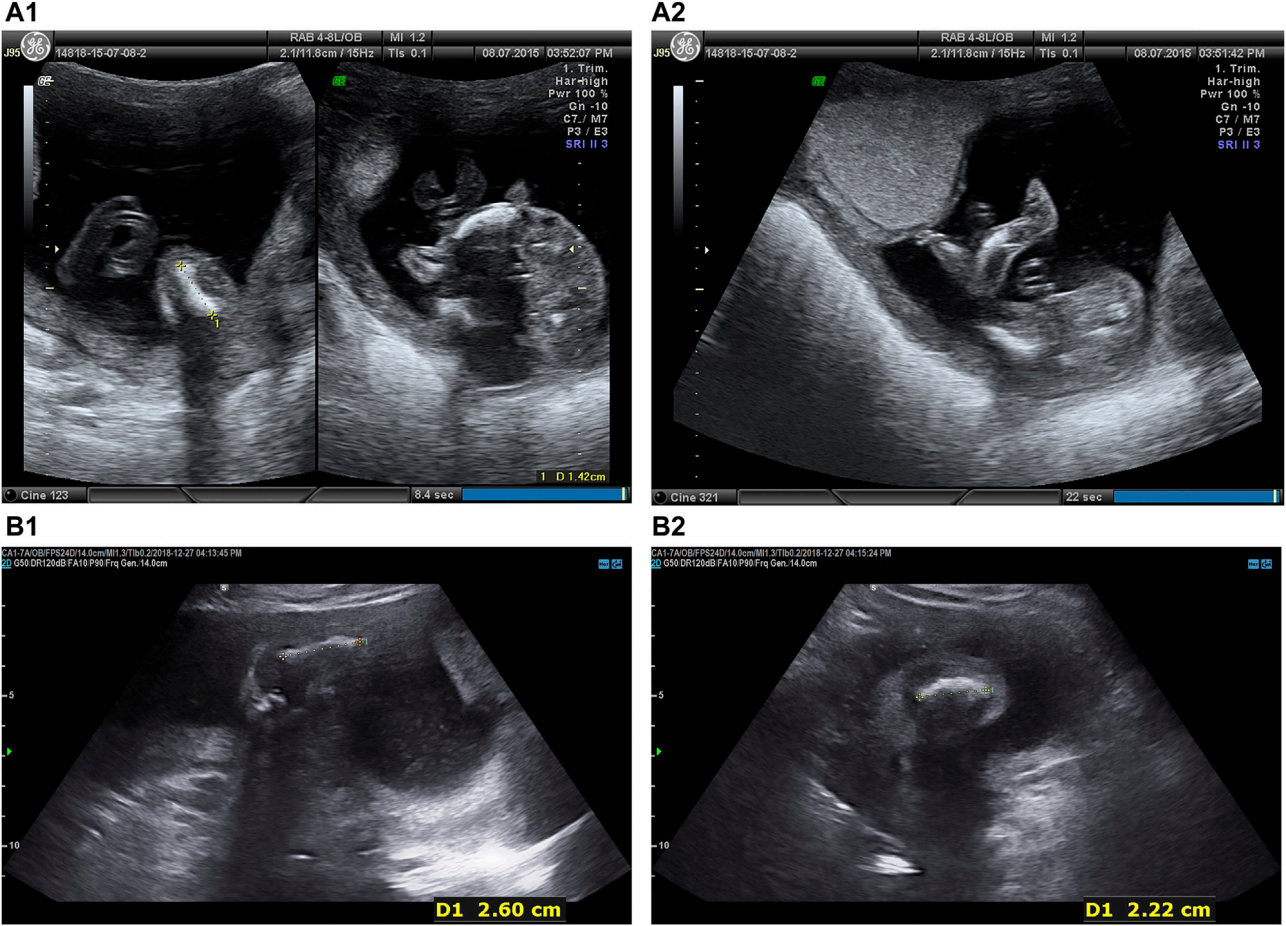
Prenatal ultrasonic examinations of the two fetuses in the second trimester showed bowed extremities (A) Intrauterine fetal ultrasonic examination of the first pregnancy at 18 + 5 weeks in 2015: navel, oval skull ring, double top diameter of 4.0 cm, lateral ventricle cerebellar hemisphere form with no obvious abnormalities, bowed lower extremities, bowed femur (1.4 cm), bowed tibia and fibula (1.4 cm), bilateral humerus (1.8 cm), radius (1.6 cm), bowed ulna (1.7 cm), crossroads of fetal heart breaks (0.2 cm), (A1 left) Femur. (A1) Tibia. (A2) Fibula.(B) Intrauterine fetal ultrasonic examination of the third pregnancy at 24 + 2 weeks in 2018: navel, oval skull ring, double top diameter of 5.6 cm, midline head circumference (20.4 cm) in the middle and lateral ventricle cerebellar hemisphere form with no obvious abnormalities, bowed upper and lower extremities, fetal bilateral bowed femur (1.8 cm), bowed tibia and fibula (1.9 cm), bowed humerus (2.6 cm), bowed radius (2.4 cm), bowed ulna (2.3 cm), fetal heart form with no obvious abnormalities, (B1) Femur. (B2) Fibula.
